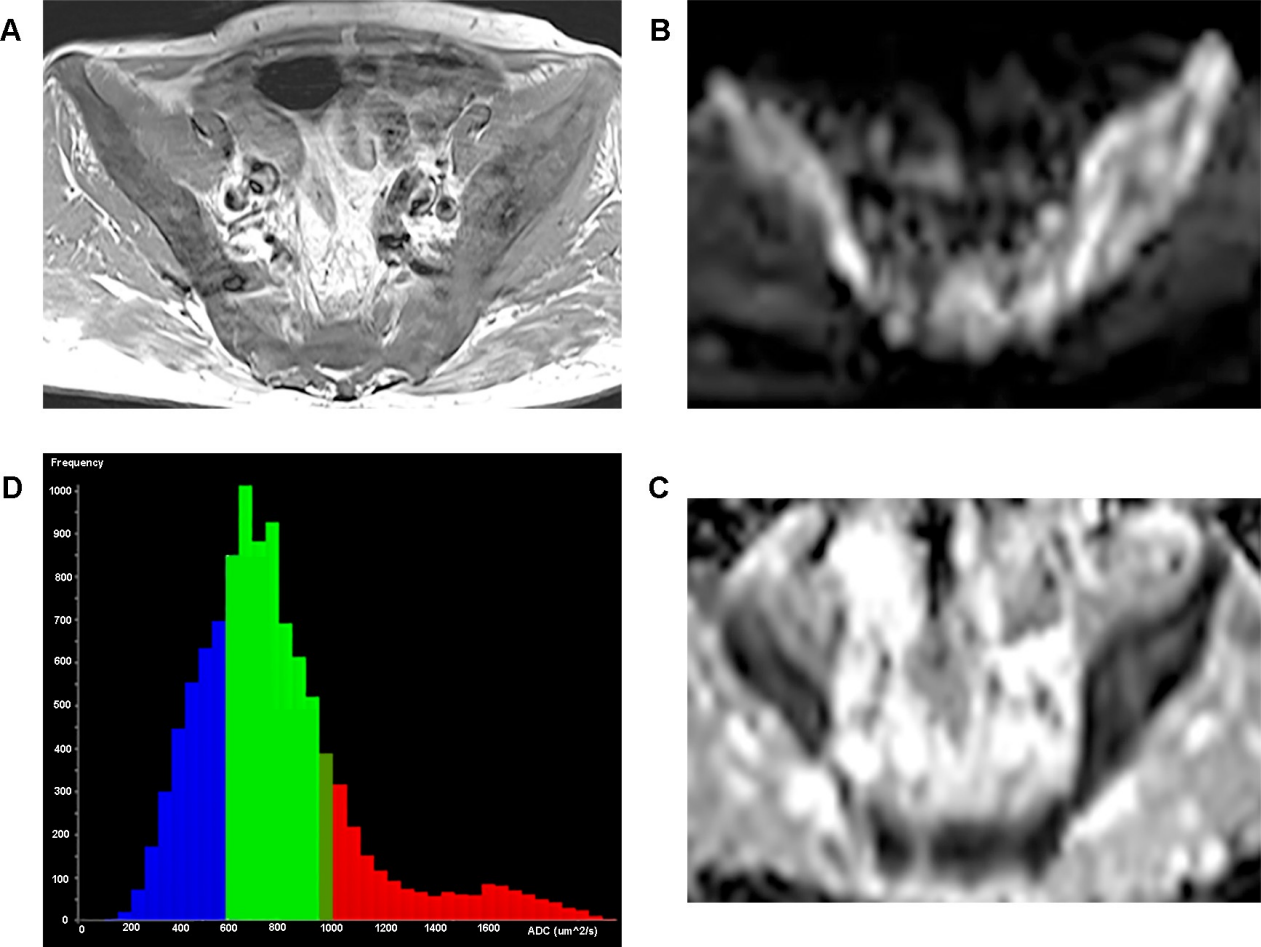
False positive case of prostate cancer. A 77-year-old man with metastasis from prostate cancer (a) Axial T1-weighted image shows diffusely hypointense signals throughout the lesion. (b) Axial DW image (b = 800 sec/mm^2^) at the same level reveals diffuse high signals in the lesion. (c) Corresponding axial ADC map (b = 0 and 800 sec/mm^2^) shows diffuse hypointense signals. Median value of ADCav was 780 ± 143 μm^2^/sec, ADCmin was 731 ± 60 μm^2^/sec. (d) In the corresponding ADC histogram of whole tumor volume, mean value of ADCvol was 767 μm^2^/sec and SDvol was 332. Both readers made a misinterpretation after adding DWI to standard MR imaging.
